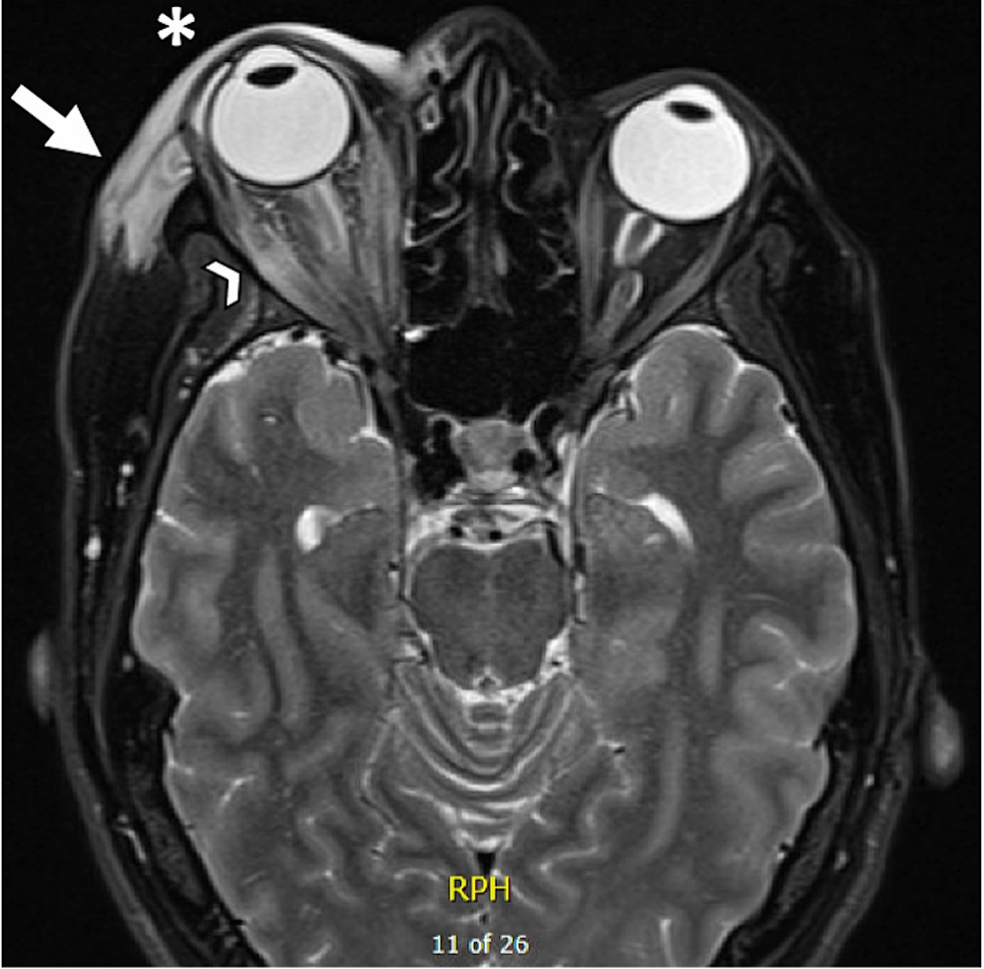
MRI orbit with contrast: T-2 weighted image showing increased enhancement and edema of the right extraocular muscles and retrobulbar fat (see arrowhead) and right periorbital soft tissues (see arrow) with associated proptosis (see asterisk). MRI: magnetic resonance imaging
